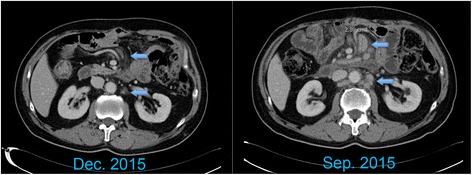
Computed tomography scan of the recurrent tumors before and after pembrolizumab treatment
